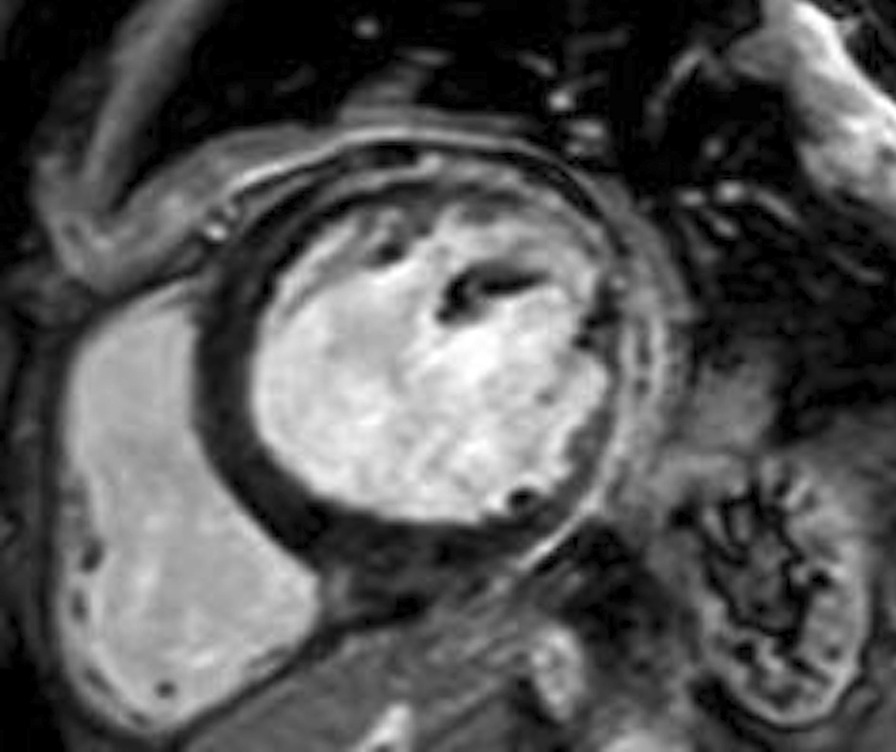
This gadolinium-enhanced cardiac magnetic resonance image shows non-homogenous late contrast agent enhancement of the thin posterolateral wall of the left ventricle. It also revealed thickening of the pleura suggestive of malignant mesothelioma
